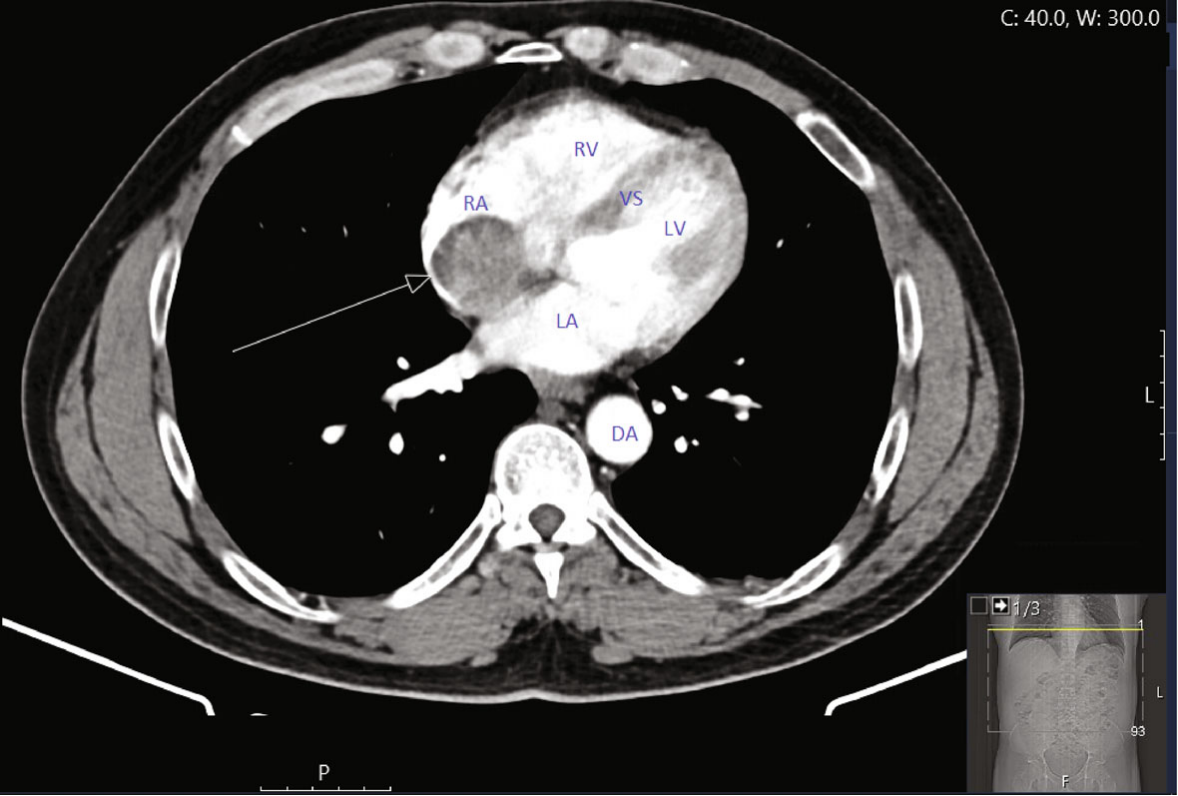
CT abdomen axial view with arterial contrast. Arrow pointing to the right atrial mass. RA = right atrium; RV = right ventricle; VS = interventricular septum; LV = left ventricle; LA = left atrium; DA = descending aorta.
